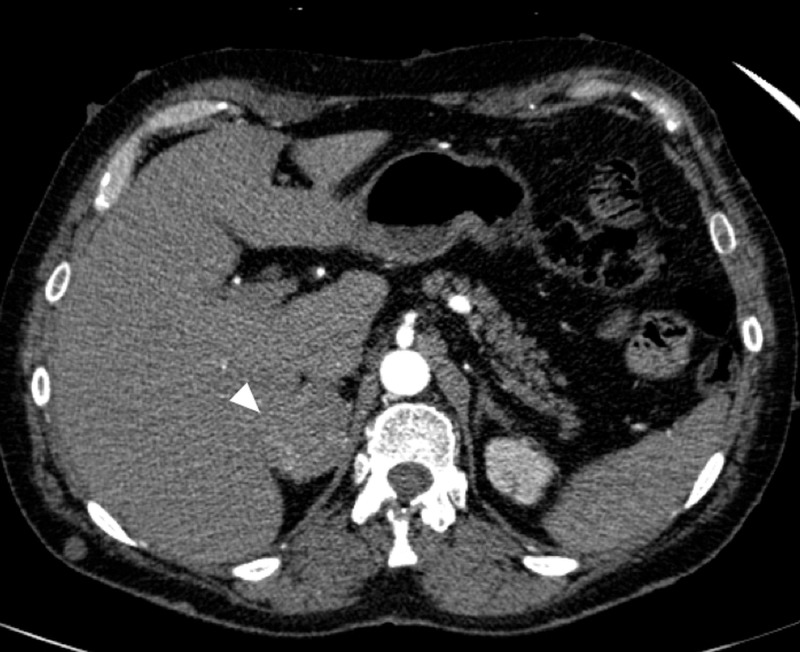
Axial contrast-enhanced CT of the upper abdomen showing the pheochromocytoma in the right adrenal gland (white arrowhead) CT: computed tomography
